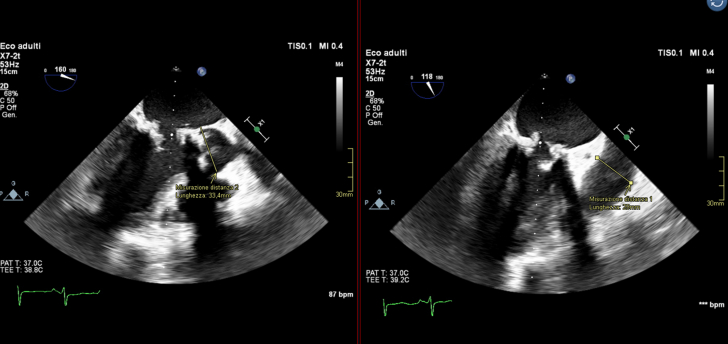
Preprocedural Screening Using TEE, Evaluating the Risk of Left Ventricle Outflow Tract Obstruction The dimensions of the aortic sinuses and the sinotubular junction were 33 mm and 29 mm, respectively, on transesophageal echocardiography (TEE). This reduced the risk of coronary obstruction.
